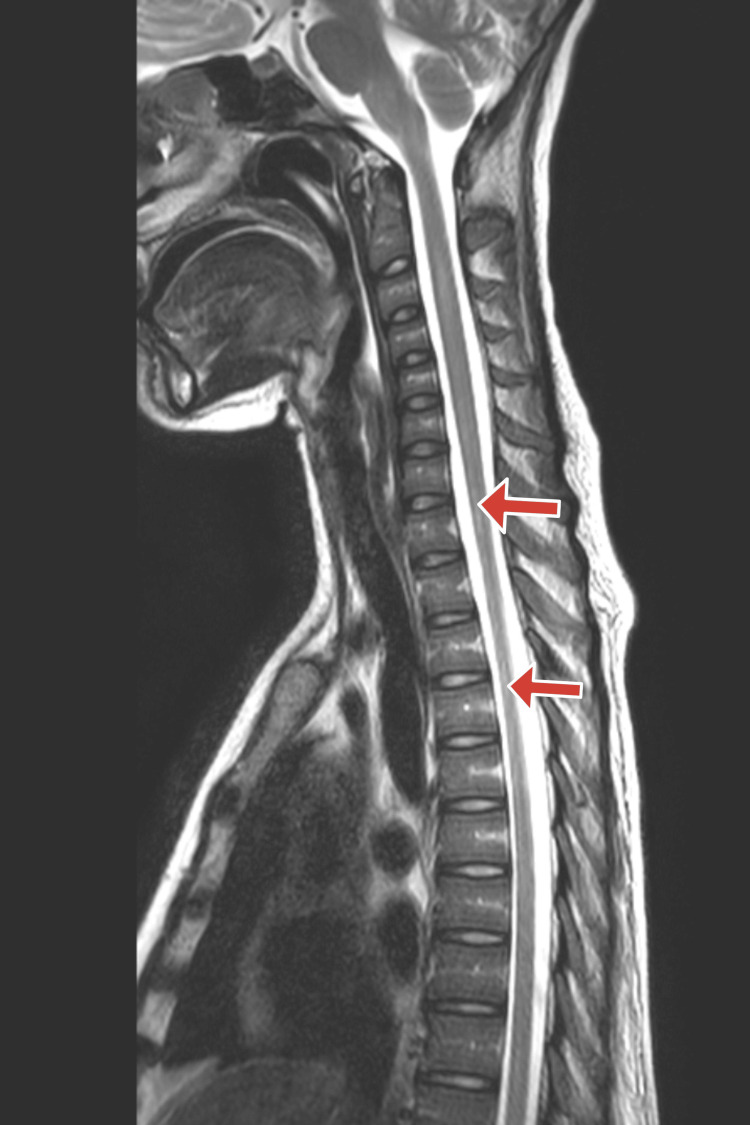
MRI spine with red arrows showing abnormal signals in the spinal cord that were bright on T2 images
